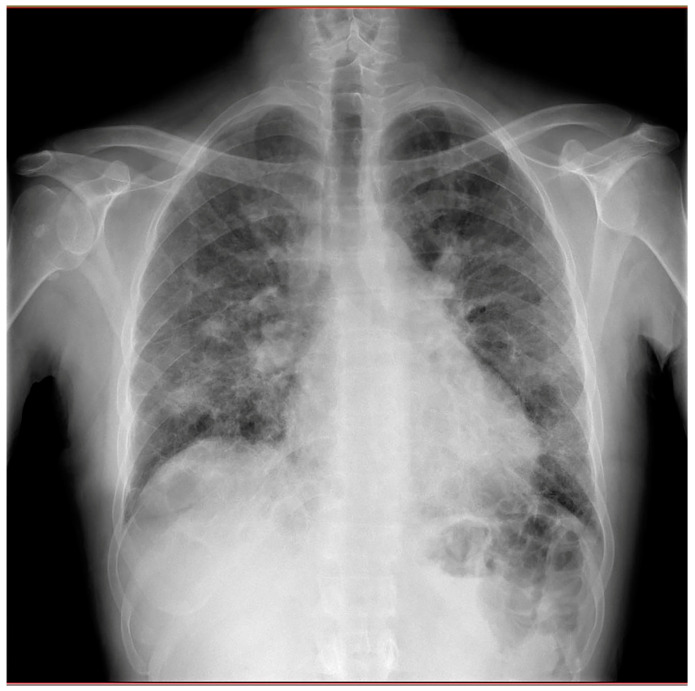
Chest X-rays revealing diffuse fibrosing interstitial lung disease with multiple bilateral parenchymal opacities, with no clear evidence of inflammatory foci.
